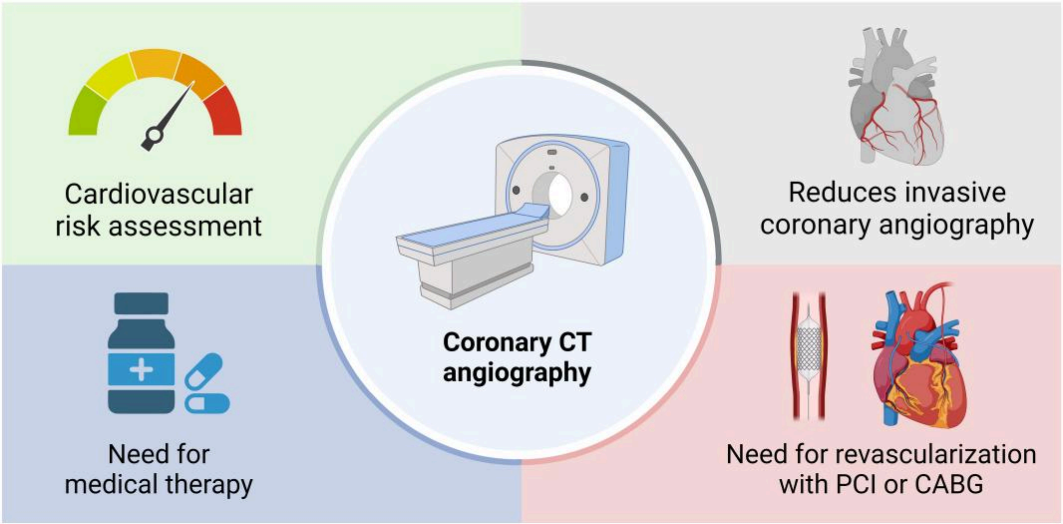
Multidimensional role of coronary computed tomography (CT) in coronary artery
